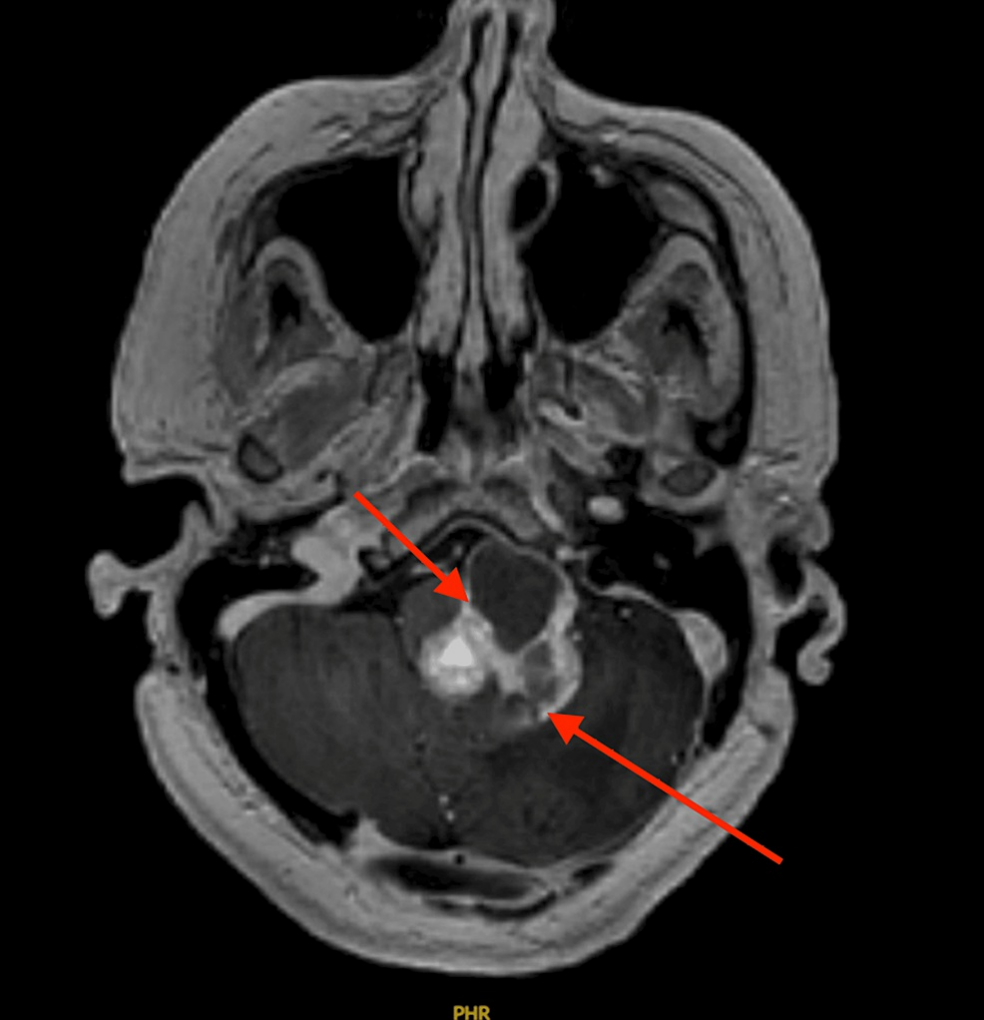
Baseline MRI prior to the new course of intra-arterial bevacizumab therapy reveals a similar appearance of the tumor now measuring 4.08 x 3.51 cm
